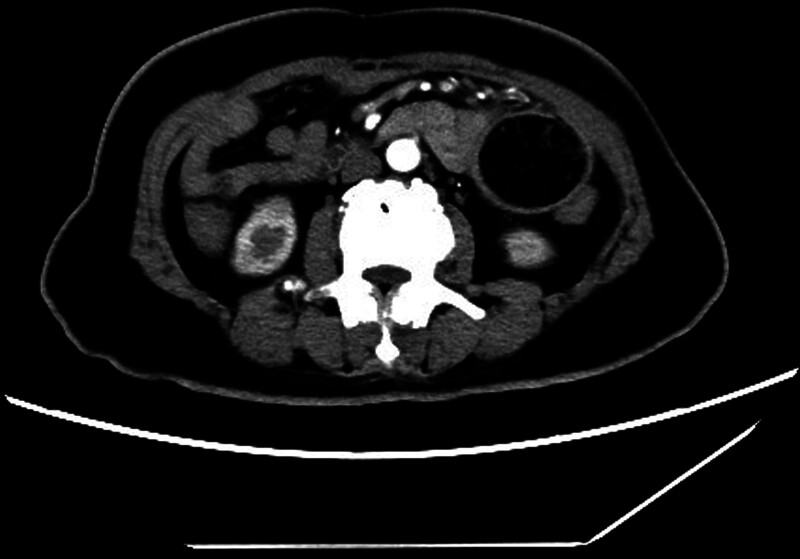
Abdominal enhanced CT: CT showed a rounded low-density shadow with sharp edges and well-defined borders in the lumen of the bowel on the left side of the abdomen, with a size of 6 cm, and no significant enhancement within the lesion. CT = computed tomography.
